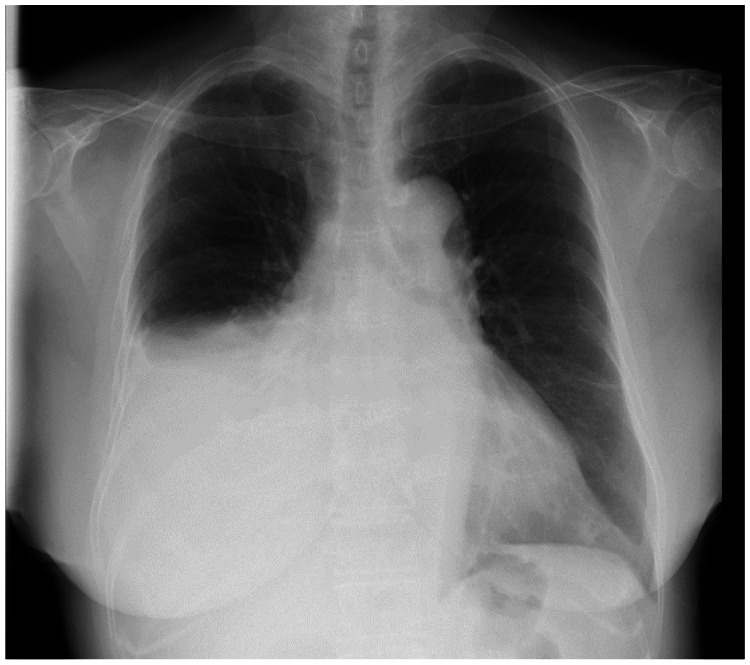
Initial chest radiography findings Right pleural effusion can be observed.
